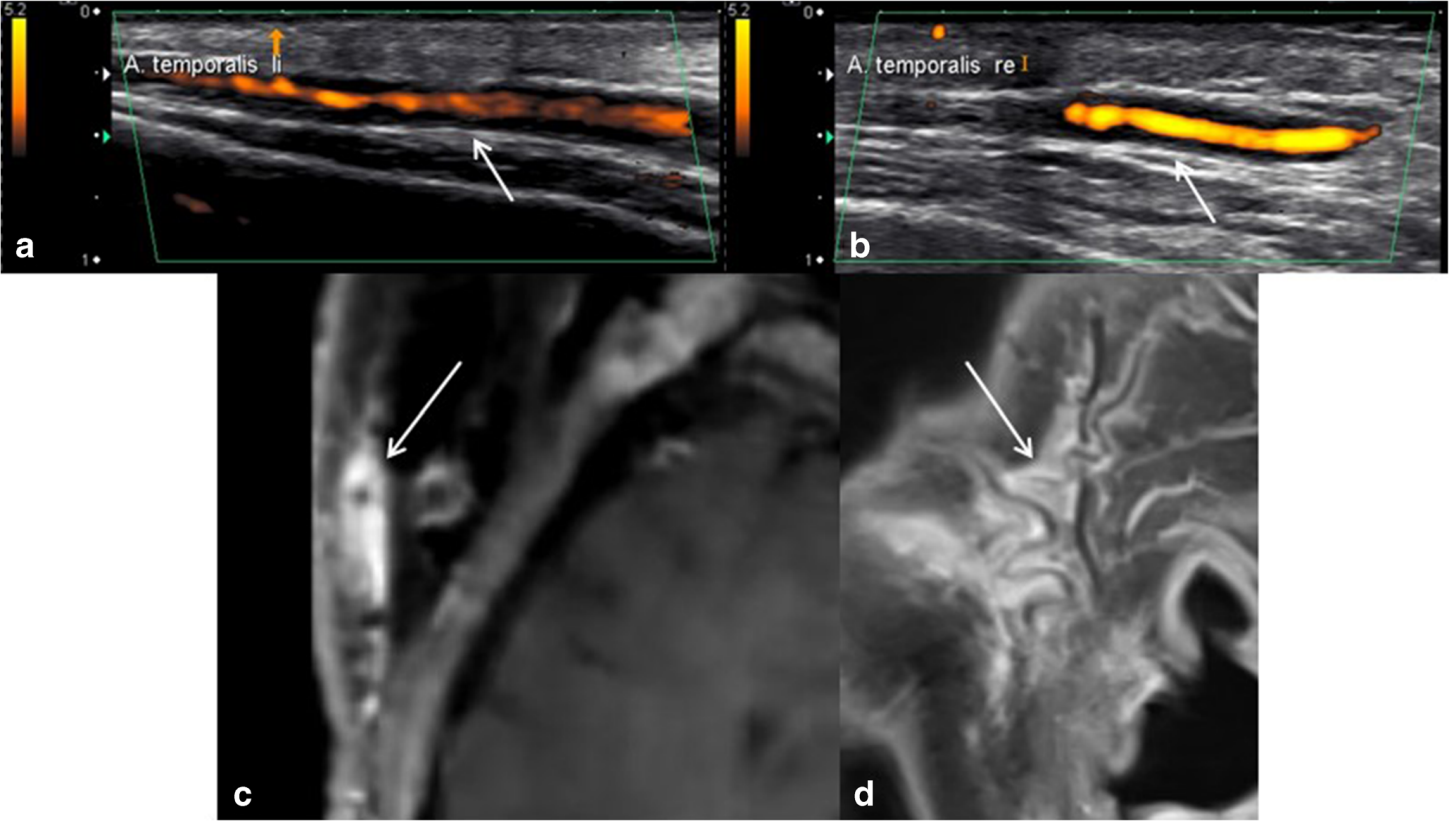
Typical imaging findings in GCA: ultrasound under compression shows a non-compressible long-segmental hypoechoic “Halo” sign around both temporal arteries, representing inflammatory vessel wall edema (a, b, white arrows). T1-weighted contrast-enhanced MRI visualizes the correlating concentric vessel wall enhancement of the superficial temporal arteries and the extended inflammatory reaction of the surrounding tissue (c, d, white arrows)
